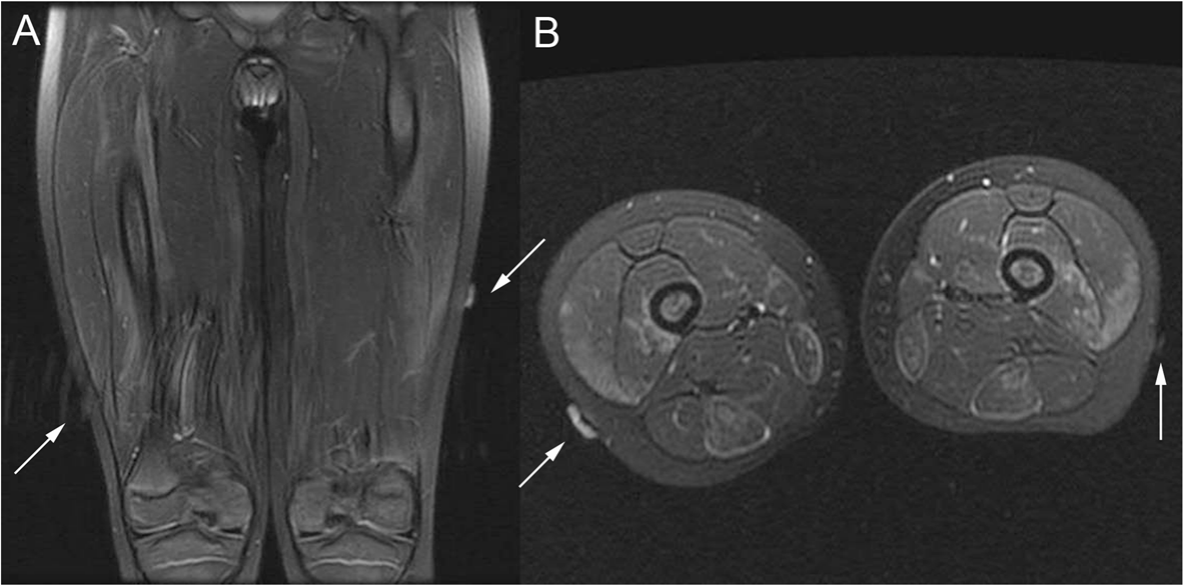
MRI of skin fiducials on lateral thighs. (A) Coronal and (B) axial T2 images show skin markers (arrows) overlying the high T2 signal within the vastus lateralis muscles bilaterally.
